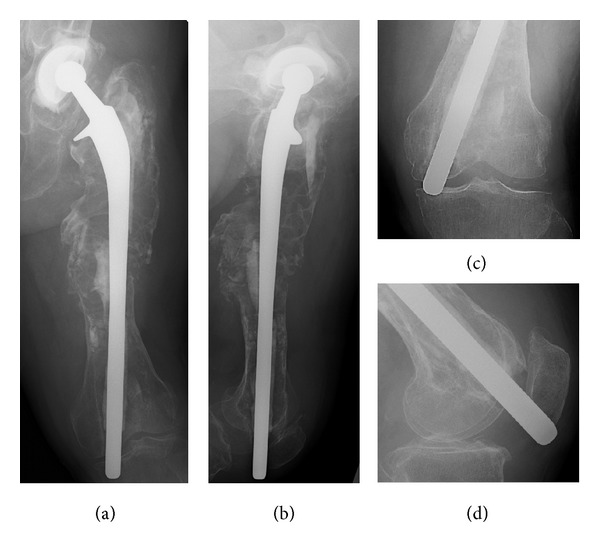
Radiographs of the left femur and knee at initial presentation. Anteroposterior (a) and lateral (b) views of the left femur 18 years after revision surgery show severe loosening of the acetabular cup and femoral stem. Radiographs of the left knee show the distal end of the stem had perforated the medial femoral condyle, reaching subcutaneous tissue through the knee joint (c), (d).
